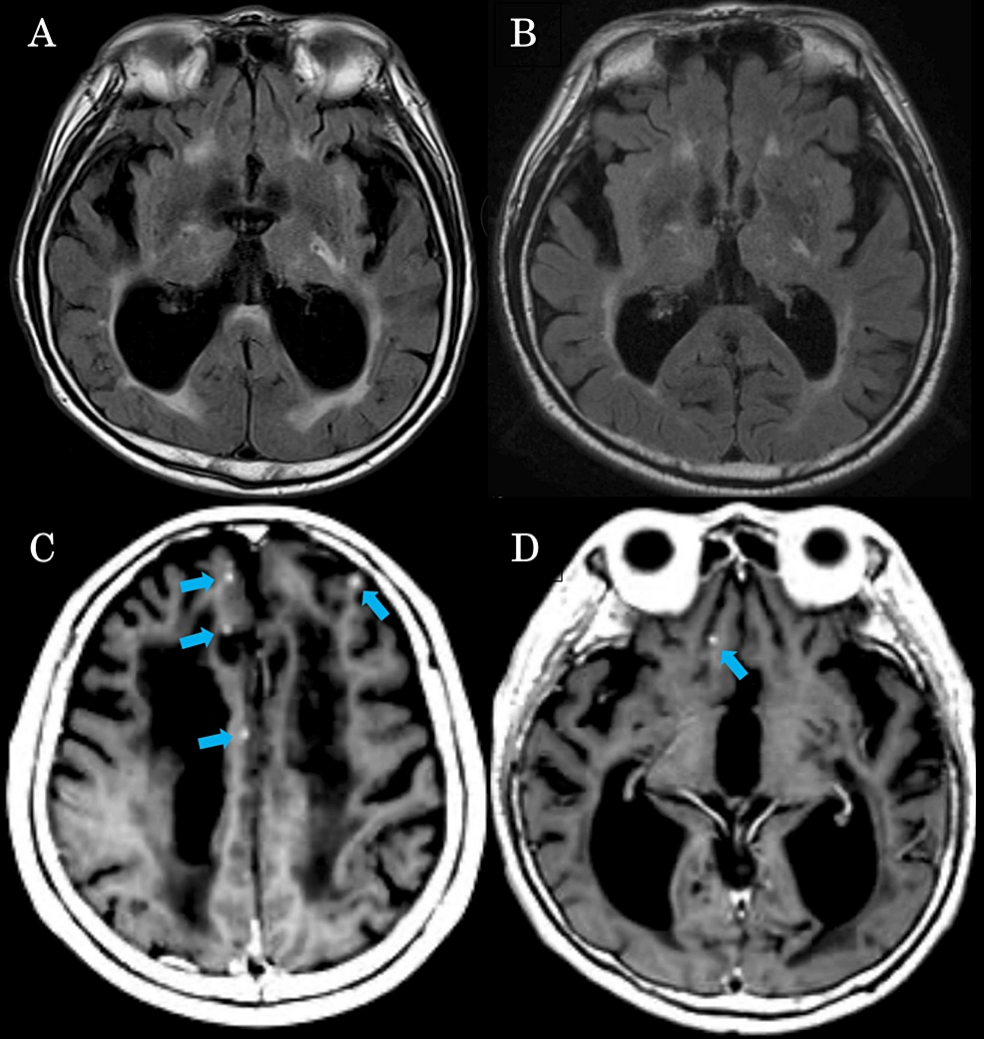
Plane brain MRI and contrast-enhanced MRI findings upon admission. Axial FLAIR brain MRI on admission shows enlarged bilateral ventricles (A) compared with axial FLAIR brain MRI seven years ago (B). Axial T1-weighted brain contrast-enhanced MRI shows multiple enhancement lesions on the brain sulci (C, D arrows). FLAIR: fluid-attenuated inversion recovery.
